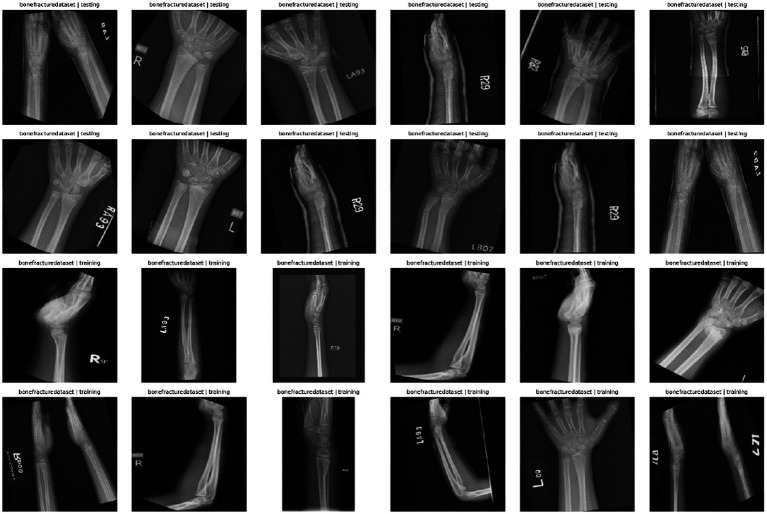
Examples of X-ray images of the bone fracture dataset. The sample images in both training and testing subsets demonstrate the differences in anatomic region, projection angle, and orientation, and acquisition conditions, and indicate the visual diversity of the dataset.
